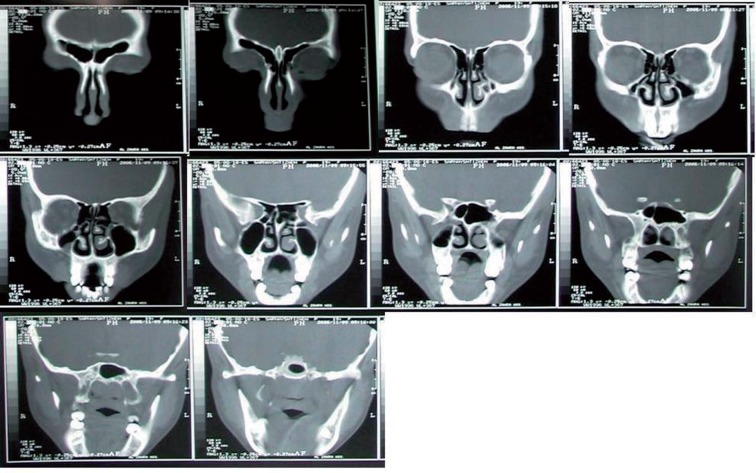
Selected coronal slices in the limited CT scan
